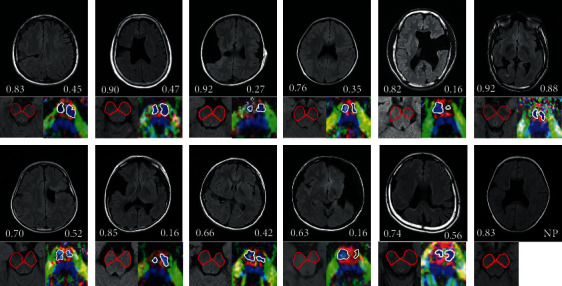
Conventional magnetic resonance imaging of schizencephaly patients and cross-sectional comparison of cerebral peduncle and corticospinal tract fibers in the pons. T2-fluid-attenuated inversion recovery (FLAIR) images of each schizencephaly patient (upper panel). Measurement of the cross-sectional area at the peduncle (lower red line) is shown in the lower left panel of each image, and the T2-peduncle symmetric index is inscribed above it. Measurement of the cross-sectional area of the corticospinal tract at the pons (lower white line) is shown in the lower right panel of each image, and the DTI-CST symmetric index is inscribed above it. NP: not performed.
